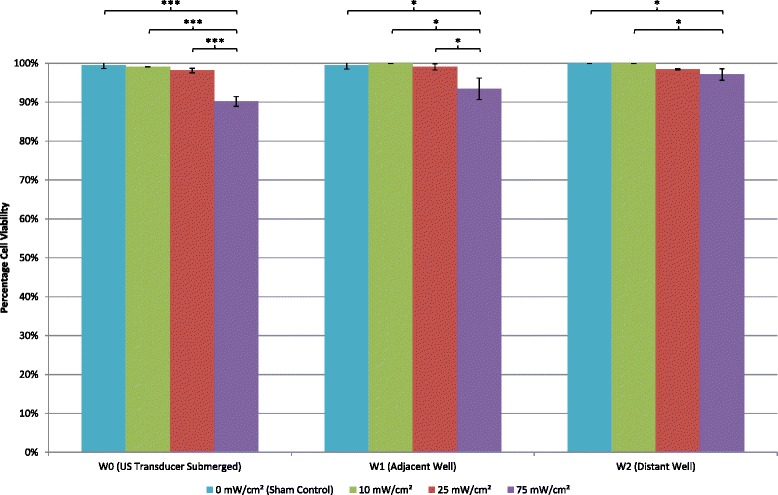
Effects of 45-kHz ultrasound on cell viability of MDPC-23 cells. Viability was determined after 8 days of culture in six-well plates with alternating days of ultrasound treatment. Three groups had ultrasound treatment each with the intensities, 10, 25, and 75 mW/cm^2^. A sham-treatment control group had no ultrasound applied to the cells. Cell viability is shown for each culture well (W0, W1, and W2), and data is expressed as a percentage of the total cell number (mean ± SD; *n* = 3). One way ANOVA statistical analysis was carried out, and the statistical significance is indicated (* *p* < 0.001;  *p* < 0.01; * *p* < 0.05)
